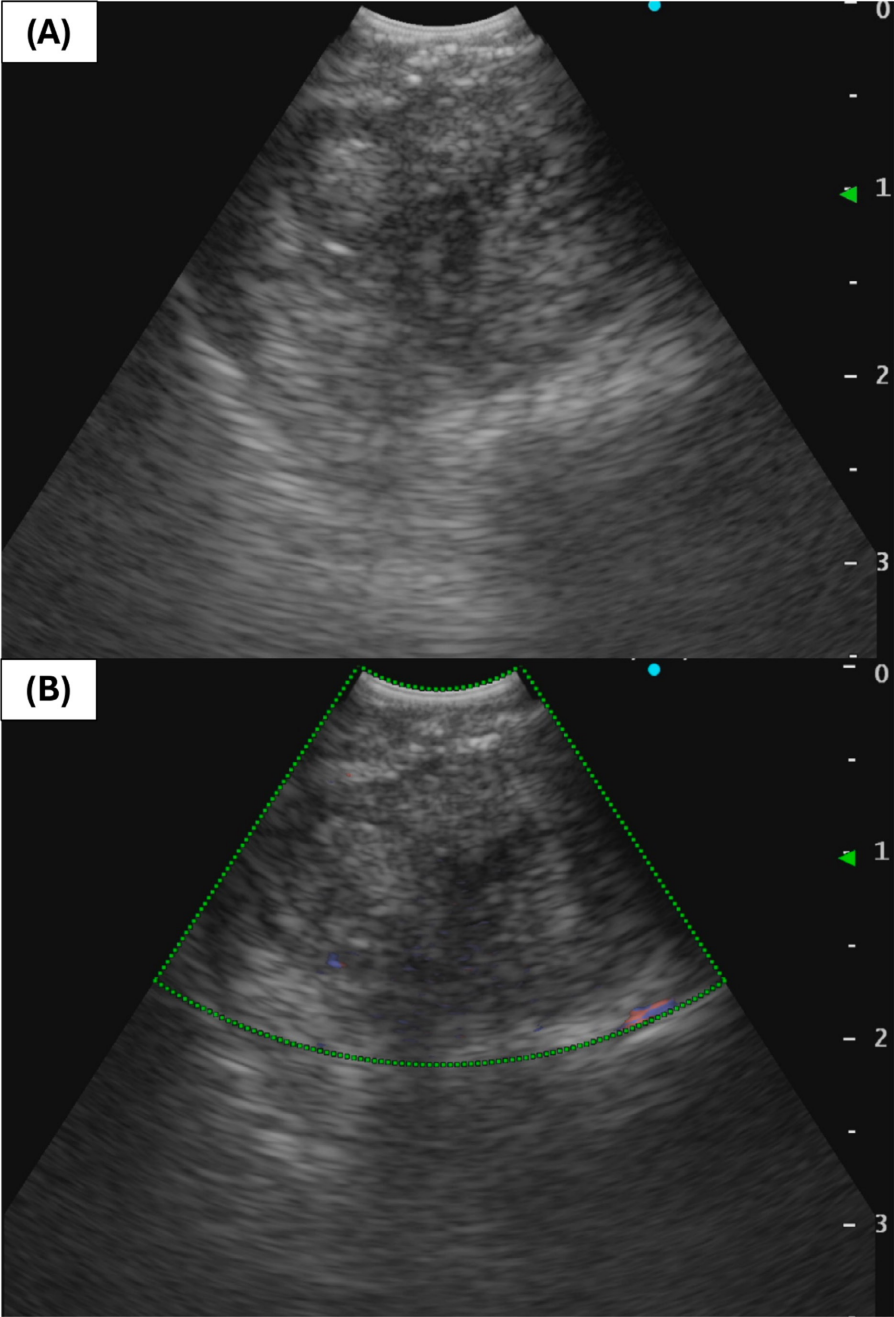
Endobronchial ultrasonography (EBUS) findings of the bronchial diverticulum. (A) EBUS revealing a hypoechoic lesion adjacent to the left main bronchus. (B) EBUS showing no detectable blood flow within the lesion.
